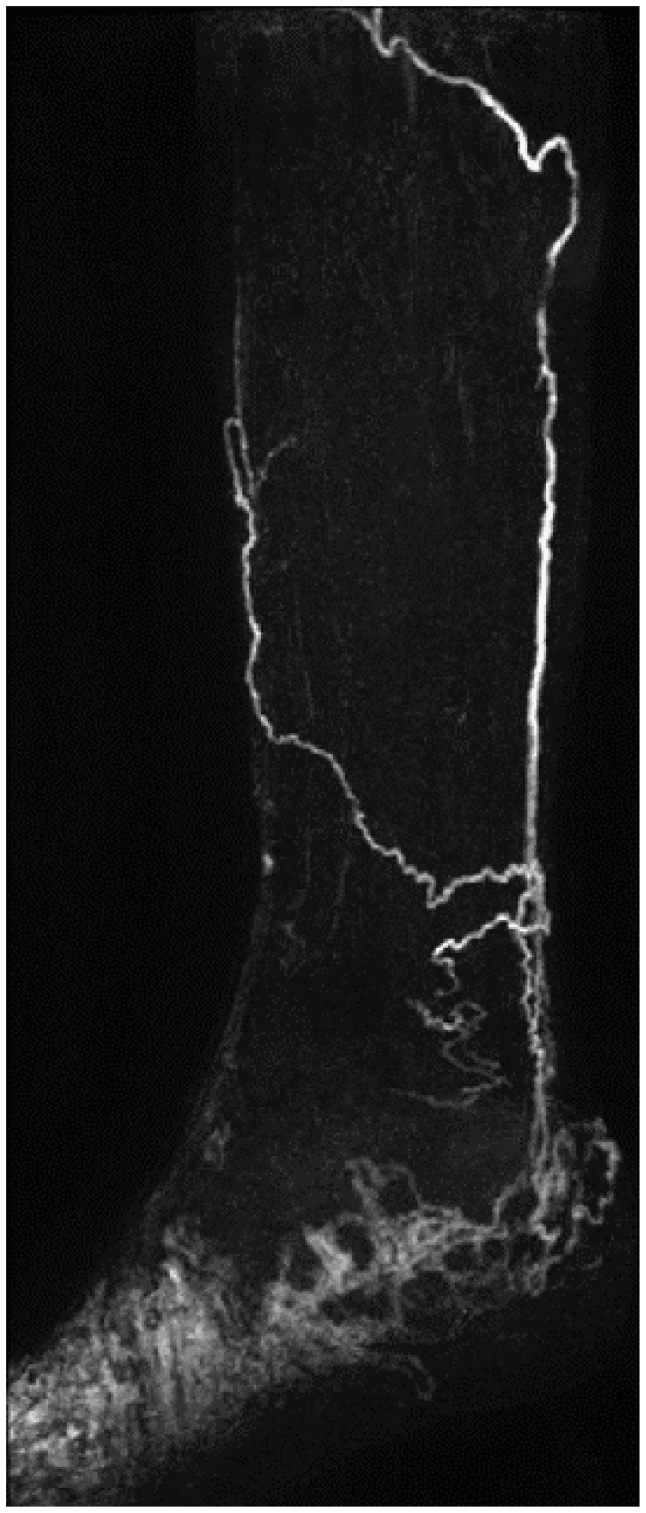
Abnormal tortuous lymphatic vessels in a lymphedema patient. Sagittal T1-weighted subtraction maximum intensity projection (MIP) for a 48-year-old man with a genetically confirmed Milroy diagnosis, demonstrating abnormal-appearing lymphatic vessels and lymphatic vessels traversing the posterior of the limb. Image obtained using interdigital contrast injection and moderately T1-weighted three-dimensional (3D) spoilt gradient echo imaging, as described in this study.
